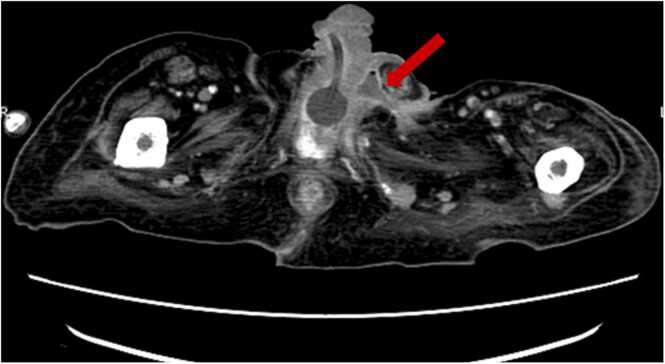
Contrast computed tomography image of the abdominopelvic region showing a subcutaneous abscess connected to a urethrodermal fistula (red arrow).
